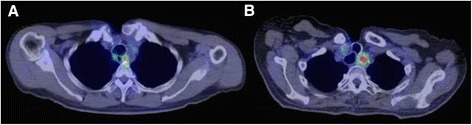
PET/CT depicting increased of FDG uptake at the paratracheal site. a PET/CT in 2006. b PET/CT in 2012
